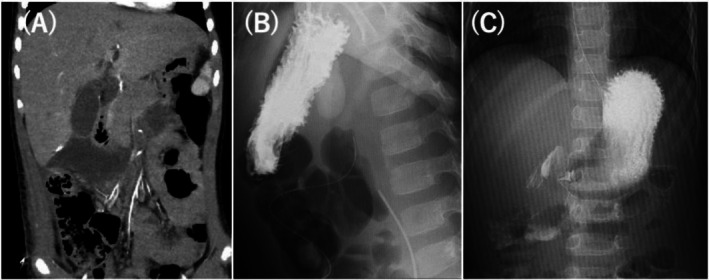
(A) Contrast‐enhanced CT on postoperative day (POD) 7. A hematoma is observed around the duodenum, causing duodenal obstruction. (B) Gastrointestinal contrast study on POD 7 was performed in a right complete lateral decubitus position with the duodenum positioned directly below the stomach; and manual abdominal compression was applied. No contrast medium was seen passing beyond the duodenum. (C) Gastrointestinal contrast study on POD 16. In the supine position, without compression, contrast medium is observed flowing beyond the duodenum. CT, computed tomography.
